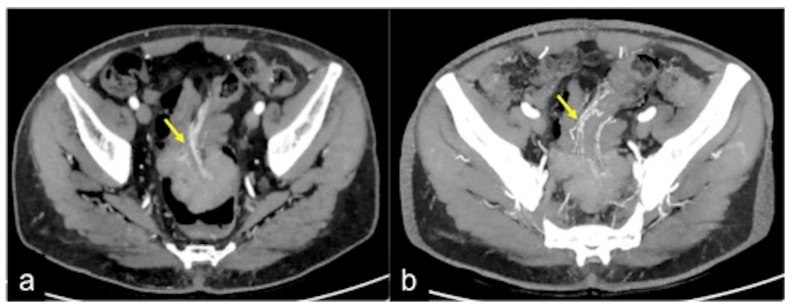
CTA multiphasic study in the arterial phase (a) and MIP reconstruction (b); an invaginated polyp in the sigmoid colon with arterial vascular support is visible (a,b, arrow).
